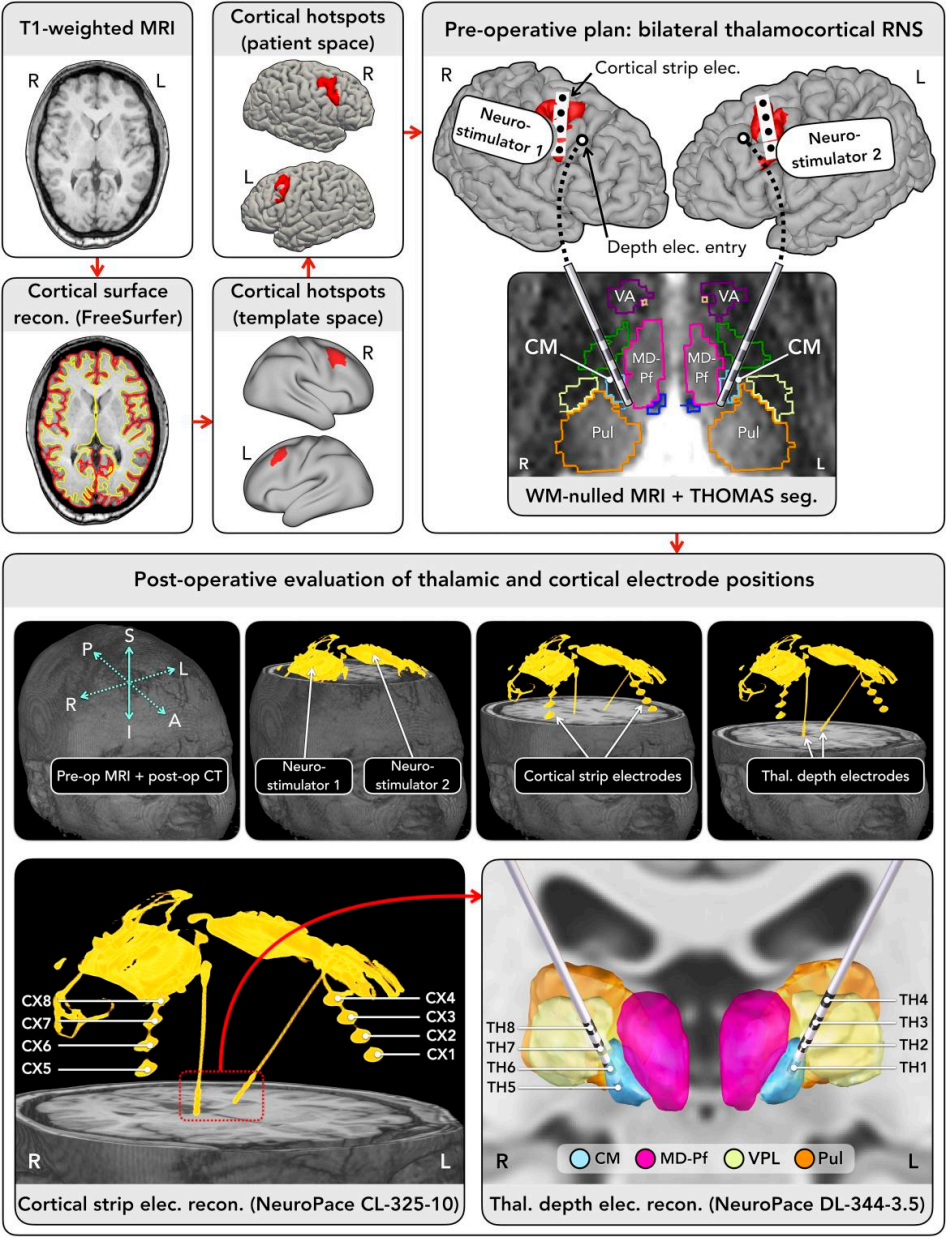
Preoperative plan for bilateral thalamocortical RNS System implantation and postoperative evaluation of electrode positions. The preoperative plan involved warping the location of the cortical hotspot from a template brain space (FreeSurfer’s ‘fsaverage’; https://surfer.nmr.mgh.harvard.edu/fswiki/FsAverage)^32^ to each patient’s native brain space. The location of the hotspot in native patient brain space was used to guide placement of the cortical strip lead on each brain side. Thalamic depth lead positions were planned using direct targeting of the centromedian nucleus, as seen on each patient’s white-matter-nulled MRI scan,^35^ aided by an atlas-based segmentation of the thalamus performed using THOMAS software.^33^ Entry points for the thalamic depth leads were typically located anterior to the cortical leads. Postoperative CT co-registered to the preoperative MRI was used to evaluate electrode positions. Abbreviations: A, anterior; CL, cortical lead; CM, centromedian; CX, cortex; DL, depth lead; I, inferior; L, left; MD-Pf, mediodorsal-parafascicular; MRI, magnetic resonance imaging; P, posterior; Pre-op, preoperative; Post-op, postoperative; Pul, pulvinar; R, right; Recon., reconstruction; RNS, responsive neurostimulation; Seg., segmentation; S, superior; TH, thalamus; Thal., thalamus; THOMAS, Thalamus Optimized Multi Atlas Segmentation; VA, ventral anterior; VPL, ventral posterolateral; WM, white matter.
